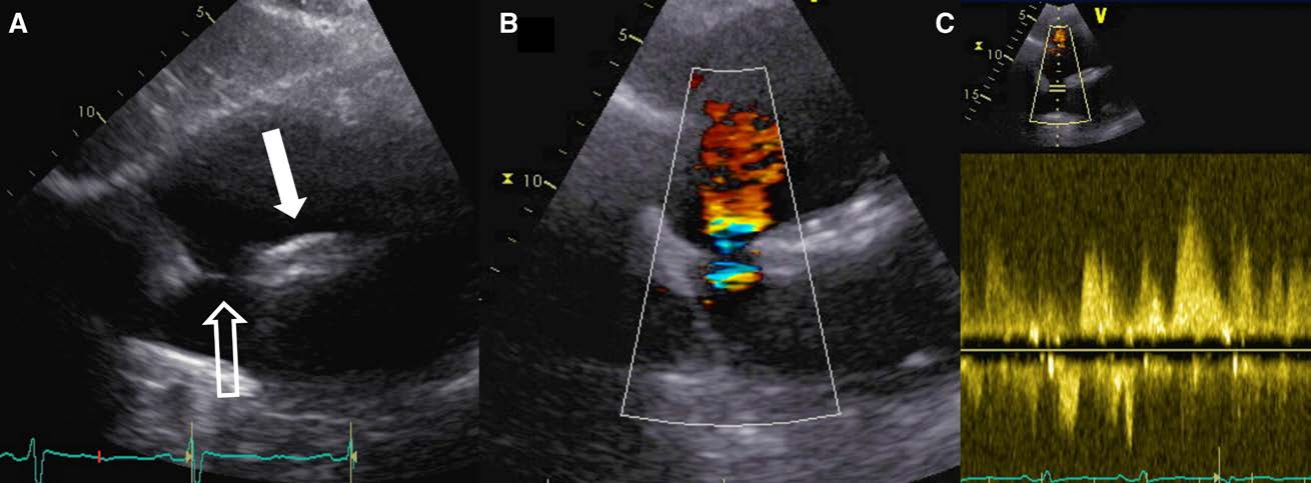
Transthoracic echocardiography after partial closure of atrial septal defects. A, Subcostal view showing the Amplatzer device (white arrow) and the persistent atrial septal defect (white bordered arrow) close to the arrival of the right upper pulmonary vein. B, Left-to-right shunting on color Doppler through the persistent atrial septal defect. C, Left-to-right shunting on pulse-waved Doppler through the persistent atrial septal defect.
